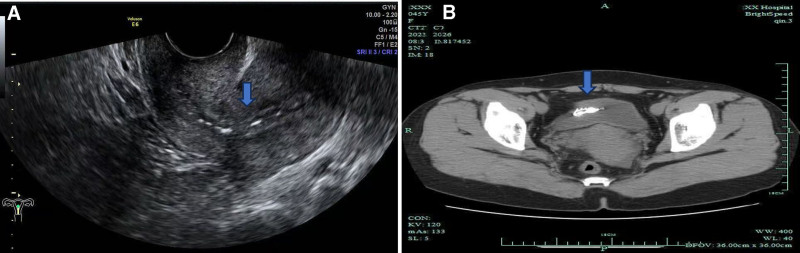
(A) Transvaginal ultrasound: intrauterine metallic focus. (B) Axial CT: abdominal IUD segment (blue arrow). CT = computed tomography, IUD = intrauterine device.
